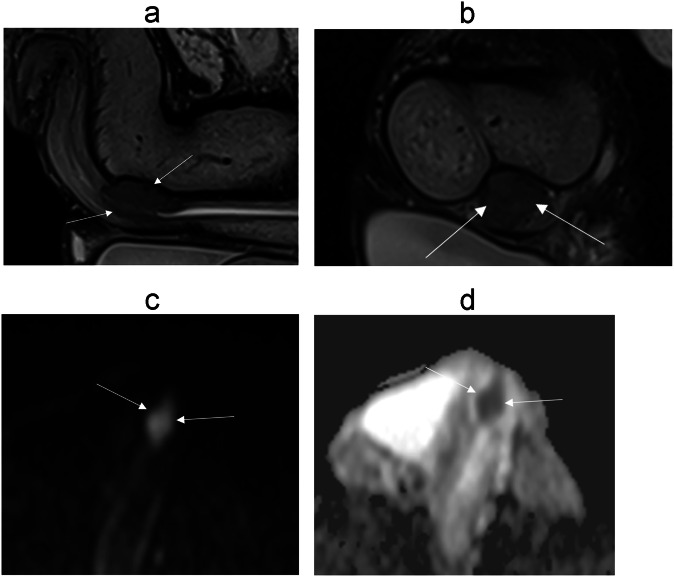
79-year-old patient referred for urethral bleeding. Penile urethral polyps on urethrocystoscopy. Extension assessment requested by MRI. a Sagittal, (b) axial T2-W MR images show a mass centered on the ureter in hyposignal T2, with invasion of the corpus spongiosum. DWI (c) and (d) ADC map shows intense diffusion restriction. Patient underwent uretrectomy in favor of an infiltrating urothelial carcinoma infiltrating the periurethral corpus spongiosum without involvement of the corpora cavernosa
